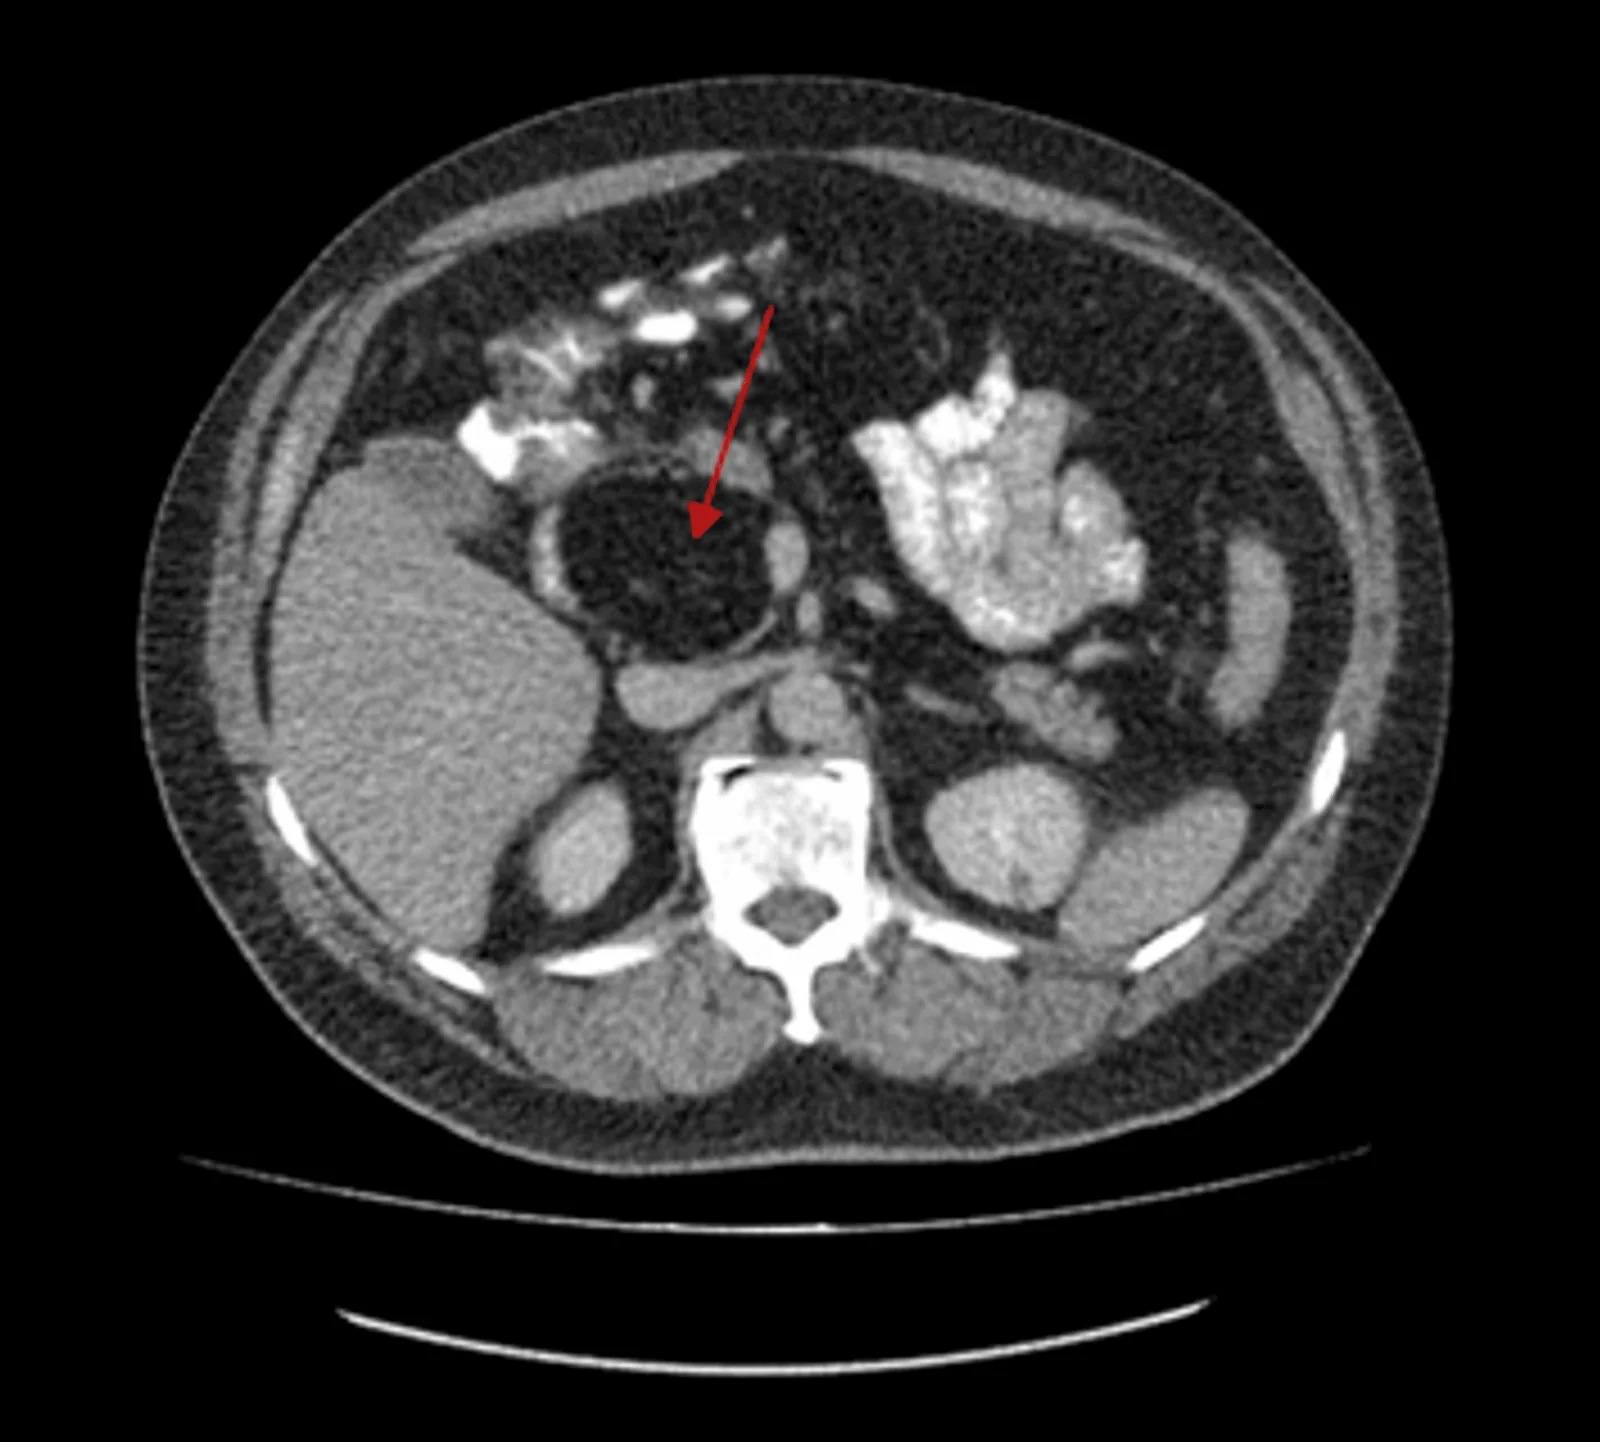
Contrast-enhanced abdominal computed tomography scan (axial view) A rounded lesion involving the pancreatic head and uncinate process, with well-defined margins and homogeneous fat attenuation (-60 HU), shows no contrast enhancement and displaces adjacent structures without evidence of infiltration (red arrow).
